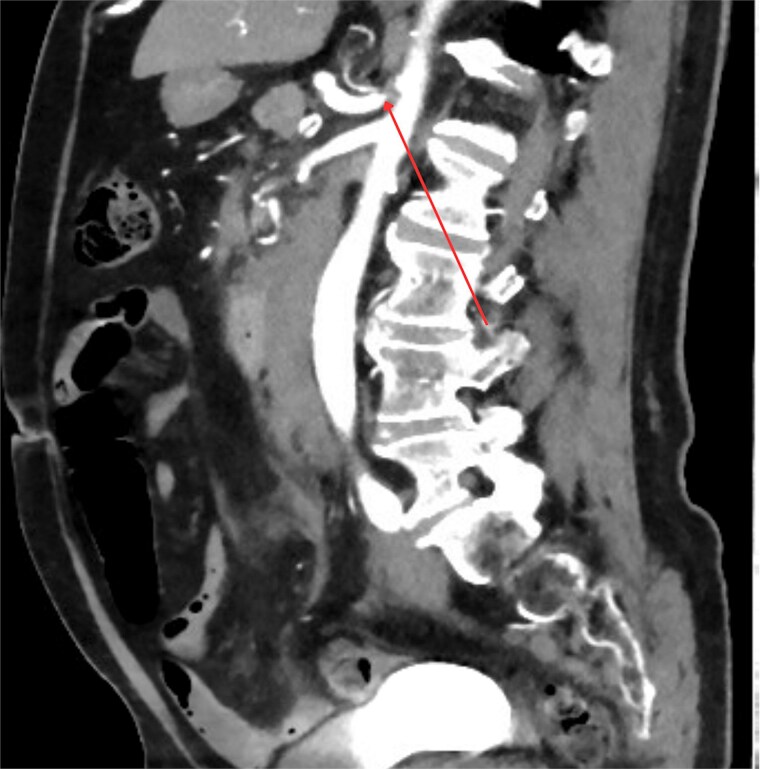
Sagittal contrast-enhanced CT image demonstrating significant stenosis at the origin of the celiac axis. The site of the narrowing is marked by arrow.
